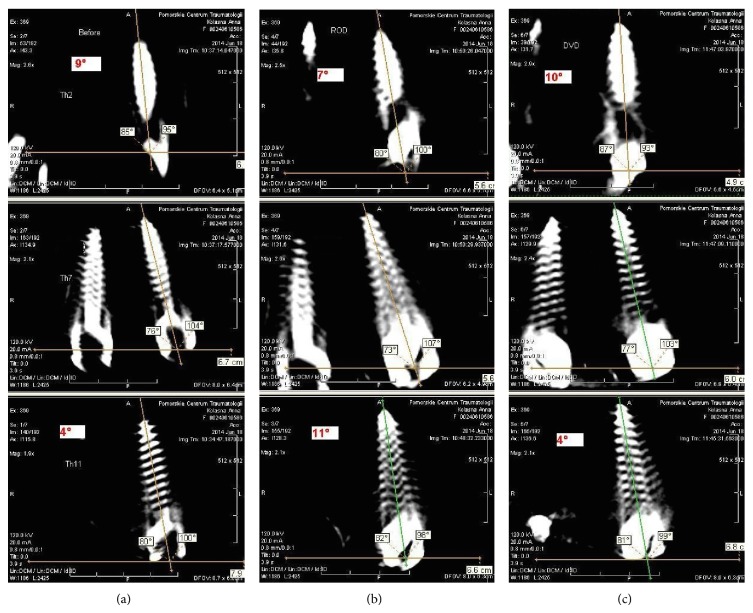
A new method of derotation assessment on the CT scans, two-step procedure. (a) Before correction, (b) after rod derotation, and (c) after DVD. Upper window: upper instrumented screw (UIS), middle window: apical screw (AS), and lower window: lower instrumented screw (LIS). The longitudinal axis of the particular screw was marked which crossed the middle of the head, the body, and tip of the screw. This line created an angle with the horizontal reference line. The difference of these angles between AS and UIS and AS and LIS before and after surgery allowed the real apical vertebral derotation (AVD) assessment. AS (°)-UIS (°): bigger windows in upper section, AS (°)-LIS (°): bigger windows in the lower section.
